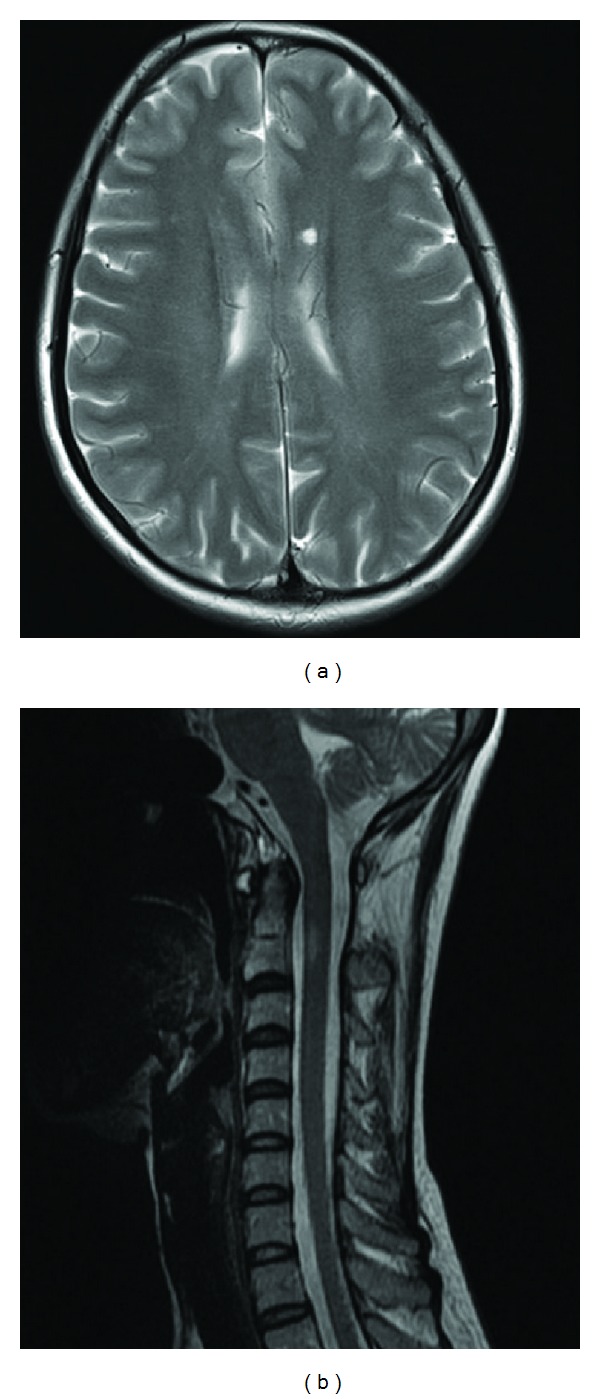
Brain MRI (a) and cervical spine MRI (b).
